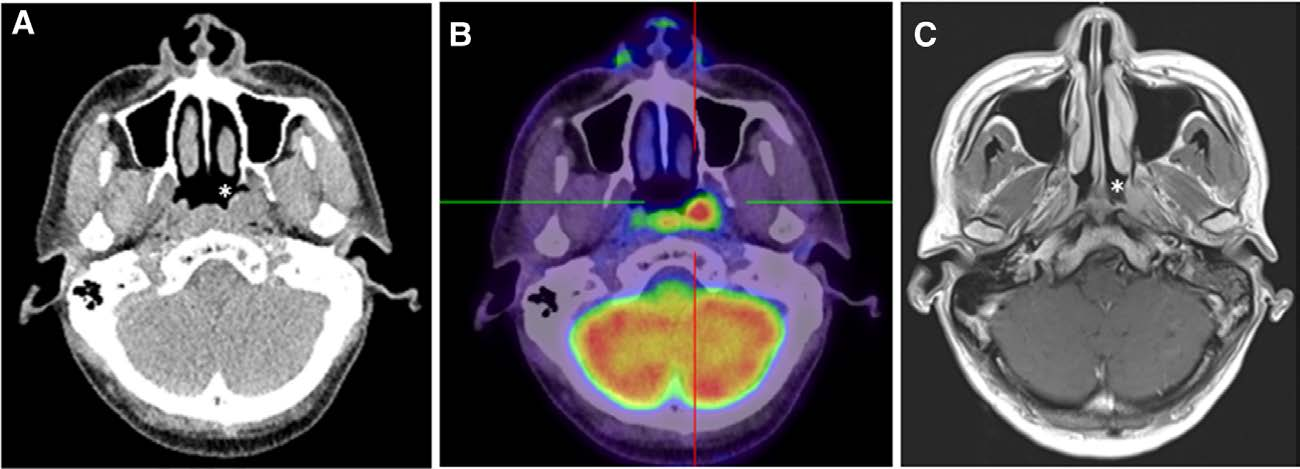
Radiological pictures of the hyalinizing clear cell carcinoma in the nasopharynx. Axial cuts of computed tomography (A), positron emission tomography-computed tomography (B), and magnetic resonance imaging (C) of T1-weighted image with contrast. The asterisk indicates the tumor. In (B), a bulge tumor with intense fluorodeoxyglucose uptake in the left nasopharynx with (1.2 cm, SUV_max_: 14.5) was noted. In (C), the axial cut demonstrates the lateral extension of the tumor to the Eustachian tube.
